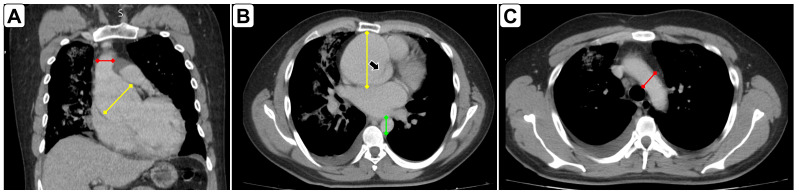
Confirmation of A-AD by chest computed tomography with intravenous contrast medium. A. Coronal plane showing a dilated aortic root/ascending aorta (continuous yellow line) and a normal aortic arch (continuous red line). B. Axial plane showing a dilated ascending aorta (continuous yellow line) with an intimal flap indicated by the arrow, and the normal descending aorta (continuous green line). C. Axial plane at the level of the normal aortic arch (continuous red line).
